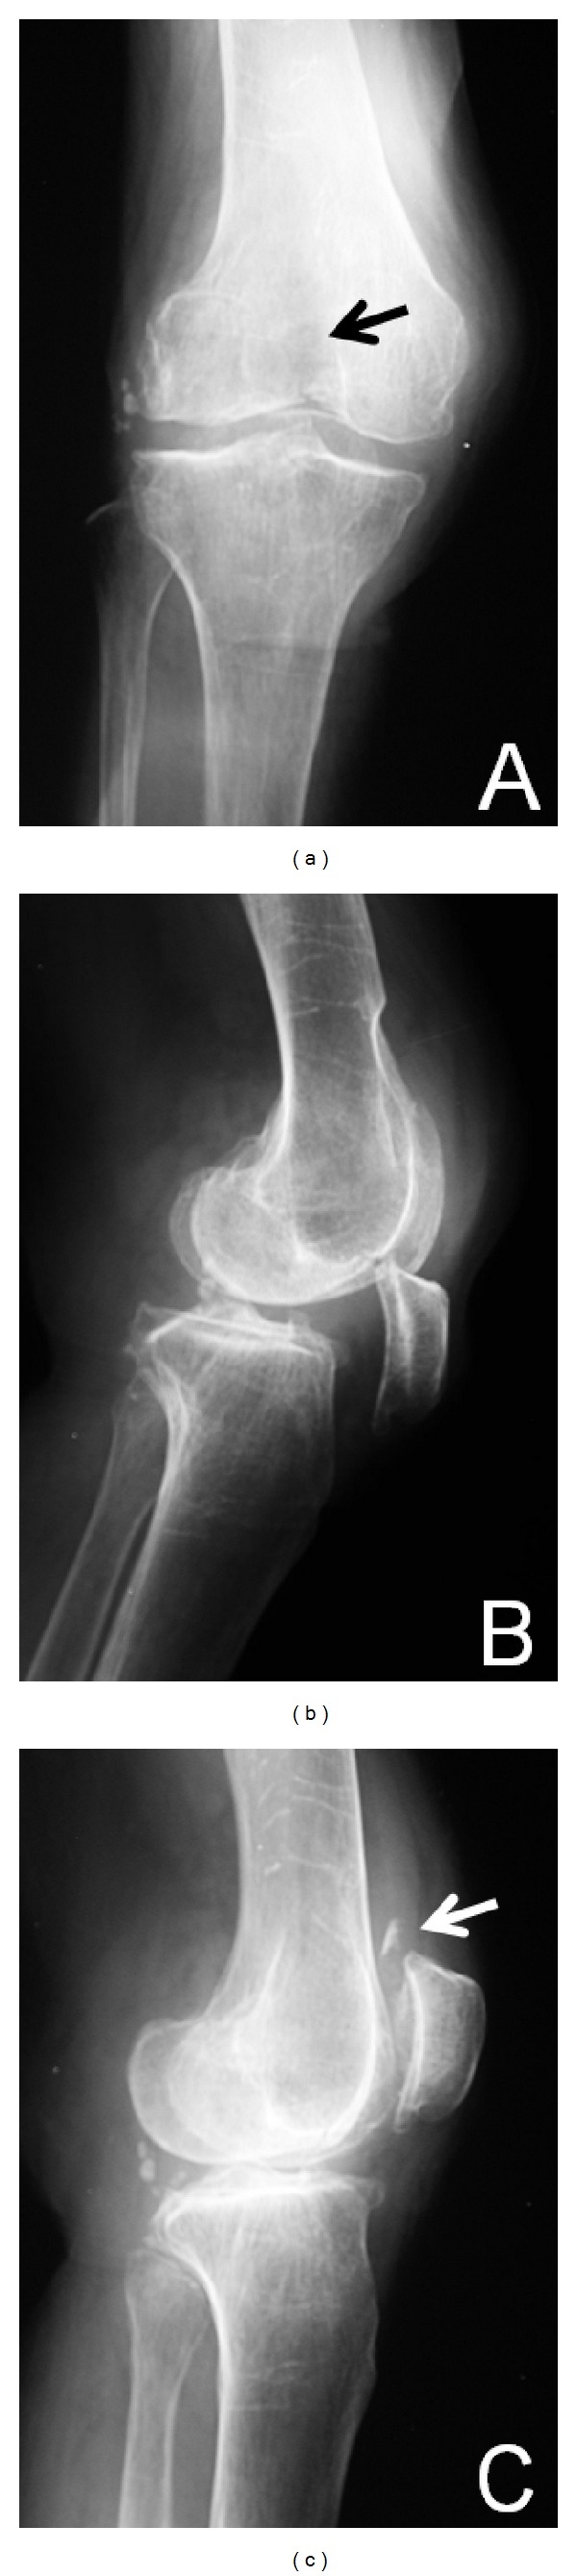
(a) Plain frontal radiography: an intra-articular fracture (arrow) is evident from the condylar fossa to the lateral condyle of the femur. (b) Plain lateral radiography: the patella is dislocated inferiorly. (c) Plain radiography after manual reduction: the remaining osteophyte and a floating bone fragment are visible (arrow).
